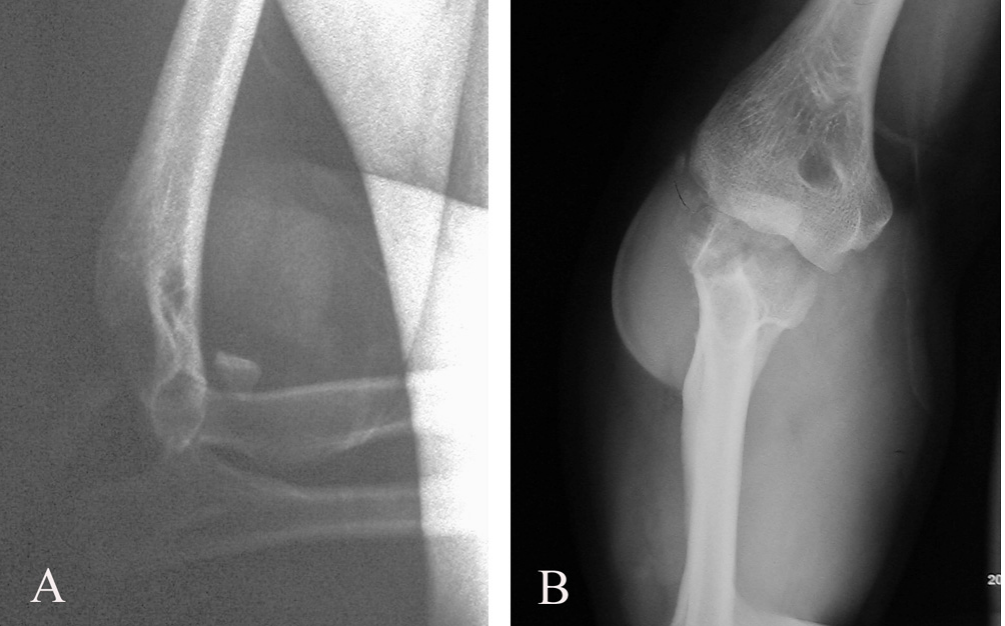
X-ray images of posterior elbow dislocation with fracture of the olecranon and radial head.
